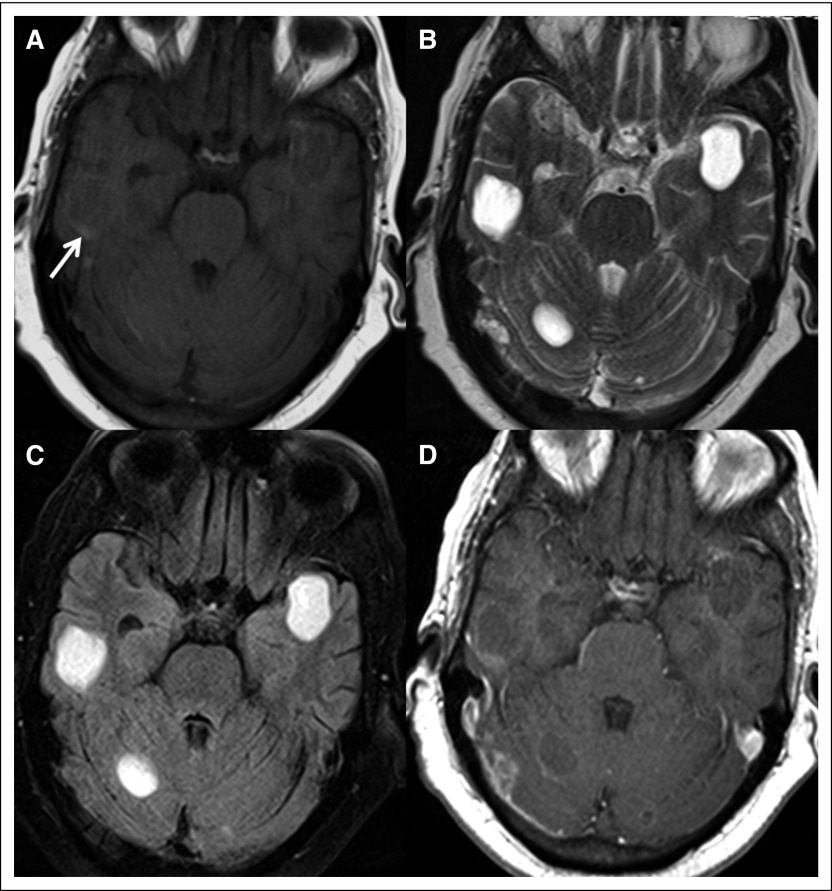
(A) T1-weighted, (B) T2-weighted, (C) fluid-attenuated inversion recovery, and (D) postcontrast T1-weighted magnetic resonance images showing multiple cystic lesions in the cerebral and cerebellar hemispheres. The cyst followed fluid signal on magnetic resonance imaging (hypointense to isointense relative to CSF on T1-weighted, hyperintense on T2-weighted, and suppressed on fluid-attenuated inversion recovery images). A few lesions showed eccentric hyperintense focus on T1-weighted imaging (arrow) that on susceptibility-weighted images revealed eccentric focal blooming corresponding to calcification seen on CT imaging; no hemorrhage was seen within. None of the lesions showed diffusion restriction or perilesional edema. Thin ring enhancement was seen in a few of the lesions.
